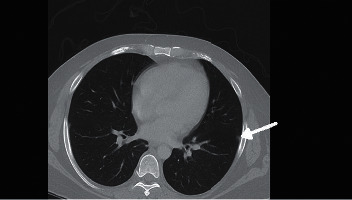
CT imaging showing multiple irregular pulmonary nodules (arrow) at different levels. The morphology of these nodules favors infectious/inflammatory nodules more than malignancy. Vasculitis can also have this appearance.
